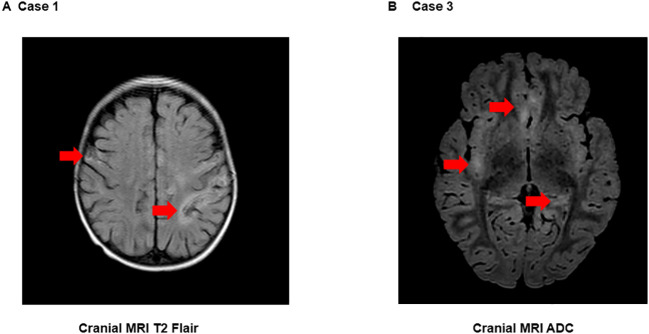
Brain magnetic resonance imaging (MRI) of Case 1 and Case 3. (A) Brain MRI of Case 1 showed multiple abnormal signals in the bilateral frontal lobes, left parietal lobe, and bilateral thalamus (as indicated by the red arrow). (B) Brain MRI of Case 3 showed multifocal abnormal signals involving the bilateral frontal lobes, insular cortices, and left temporal lobe (as indicated by the red arrow).
